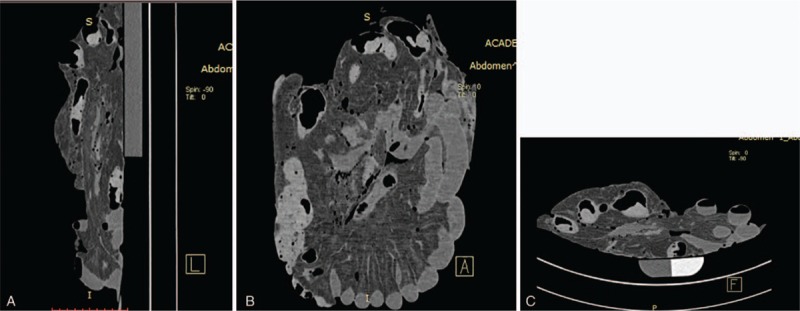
Coronal, sagittal, and cross-sectional screenshots of water-enhanced computed tomography images.
